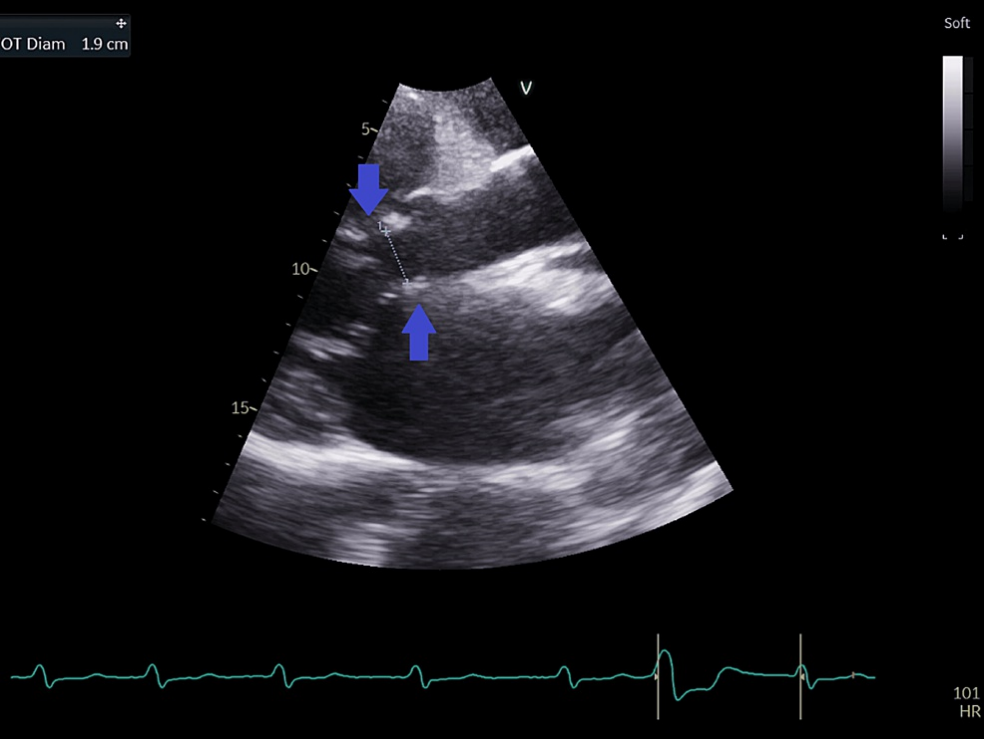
Parasternal long-axis view with measurement of the left ventricular outflow tract diameter at 1.9 cm (blue arrow).
